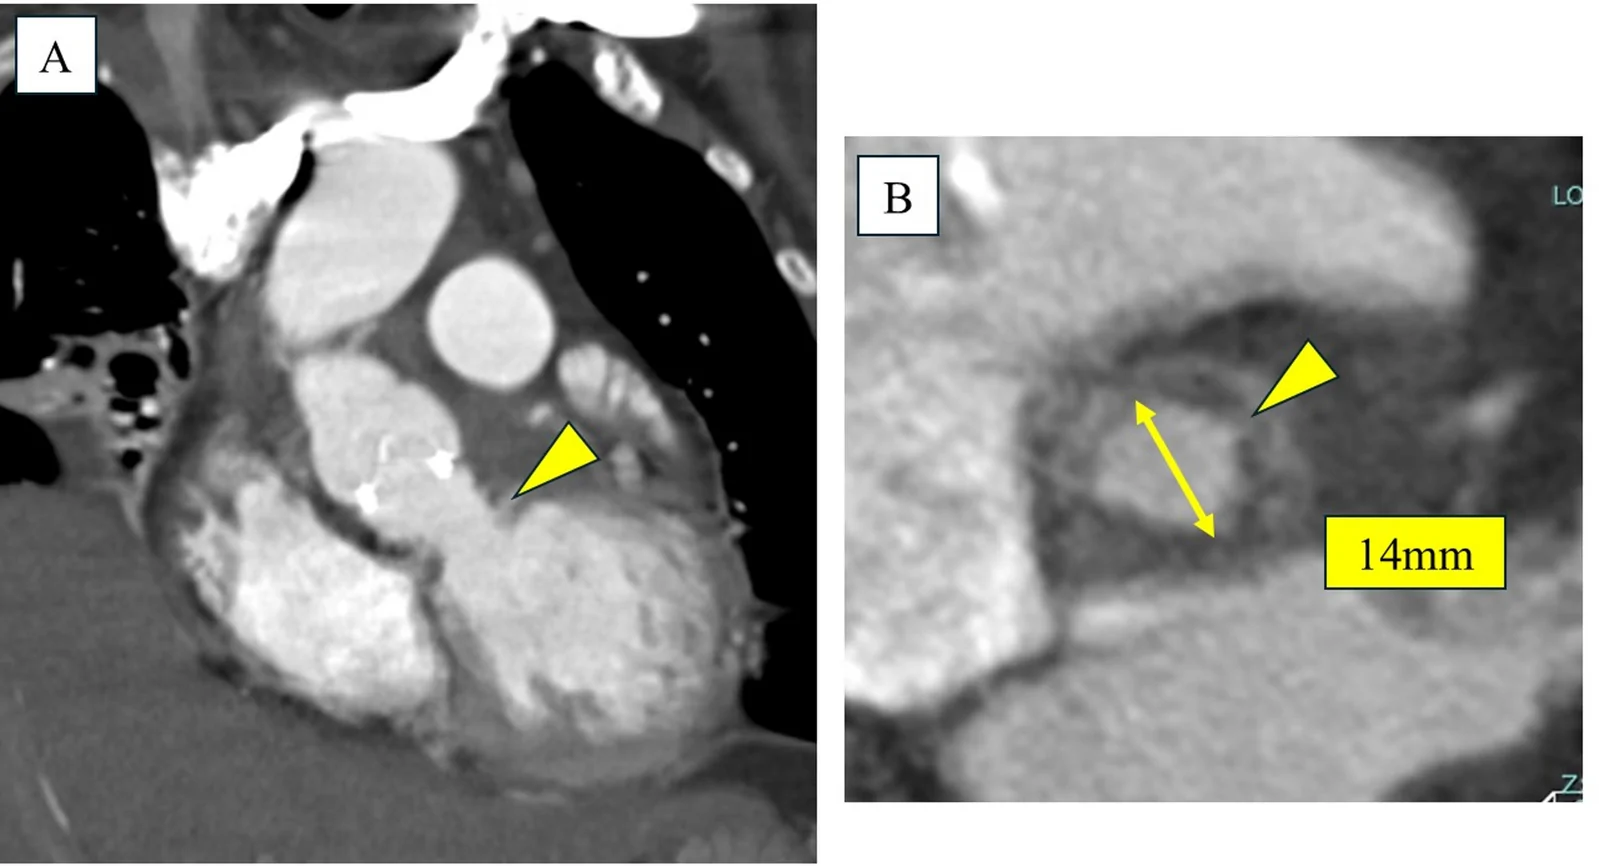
Postoperative contrast-enhanced computed tomography. A Long-axis view of the reconstructed aortic root after redo Bentall surgery using the graft insertion technique. B Short-axis view perpendicular to the left ventricular outflow tract. The yellow arrowhead indicates significant narrowing of the LVOT at the level of the inverted graft. The minimal LVOT diameter was measured as 14 mm
